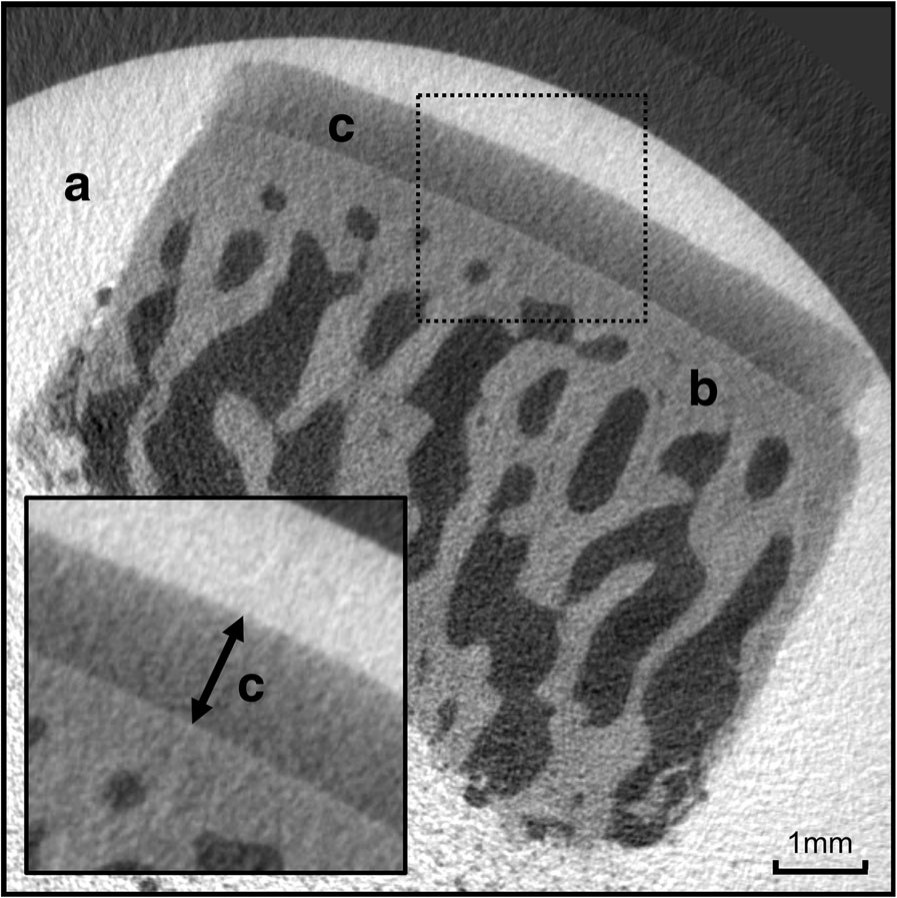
For validation of ultrasonic cartilage thickness measurement we used the micro-CT with iodine contrast agent (a) for comparison. For micro-CT measurements the cartilage-bone samples were harvested with a punch biopsy and embedded in a radiolucent tube. While the lower limit of the cartilage (c) adjacent to the bone (b) was clearly visible in the micro-CT, detection of the upper limit of the cartilage was enhanced by iodine contrast agent (a). This allowed exact determination of cartilage thickness
